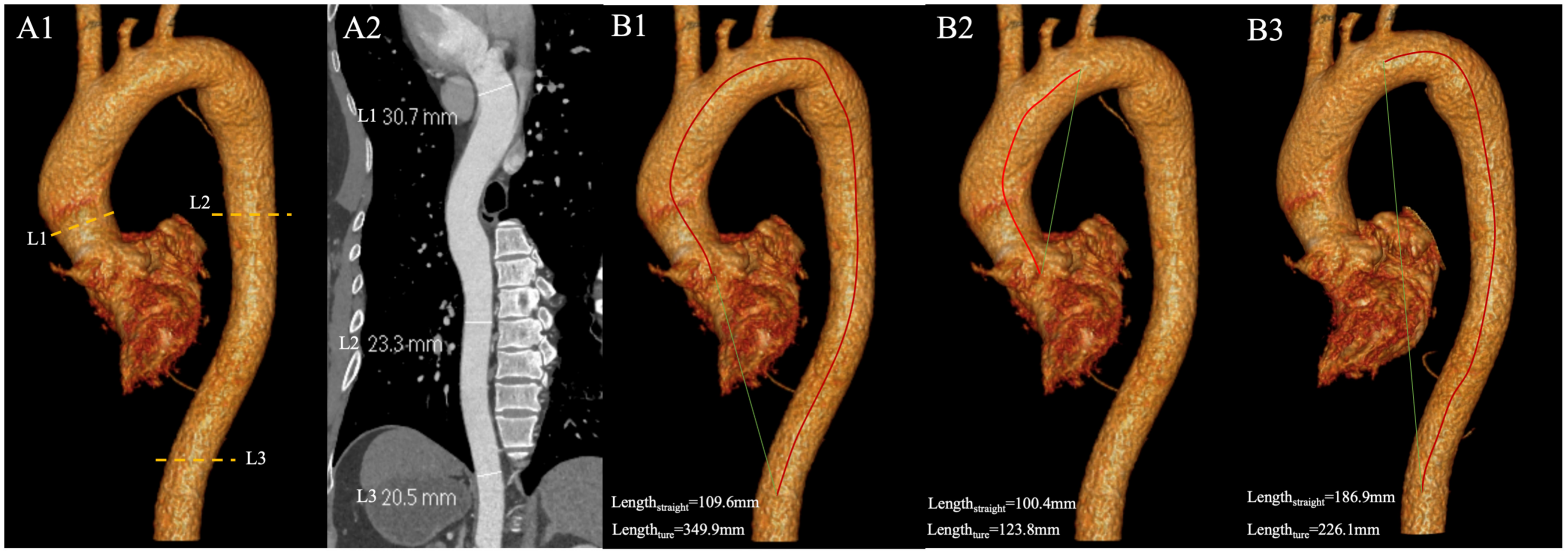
(A1) The diameter of the thoracic aorta were measured at three locations with enhanced CT images. L1 is located 1 cm distal to the sinotubular junction; L2 is in line with L1; L3 at the level of the diaphragm; (A2) Diameters of L1, L2, and L3 measured on curved planar reconstructions of the thoracic aorta; (B) Measurement of tortuosity index. The red line is the true length measurement of the vessel, and the green line is the straight-line length. (B1) The true length of the thoracic aorta is 349.9 mm, and the straight-line length is 109.6 mm. The tortuosity index of the thoracic aorta is 3.19. (B2) The true length of the ascending aorta and the aortic arch is 123.8 mm, and the straight-line length is 100.4 mm. The tortuosity index of the segment is 1.23. (B3) The true length of the DTA is 226.1 mm, and the straight-line length is 186.9 mm. The tortuosity index of DTA is 1.21. DTA, descending thoracic aorta.
